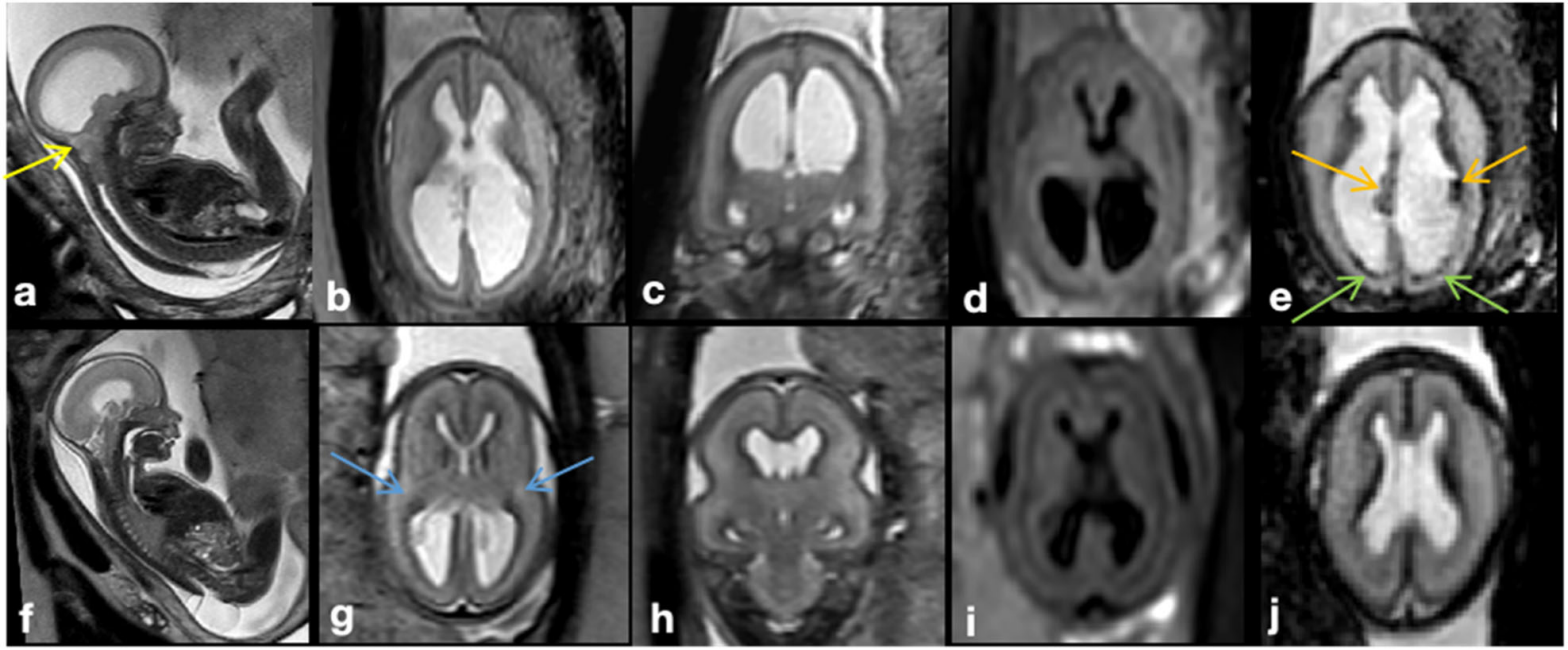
a–e MRI of a fetus with Chiari II malformation and brain edema at GW24 + 0, spinal defect formation at the lumbar level (L1/2) extending to the sacral level (S1). The posterior cranial fossa was very crowded and the vermis reached up to about C5, accompanied by protrusion of the atlantooccipital membrane (yellow arrow). b T2W-FSE images showed the compressed brain parenchyma with higher signal intensity compared to the age-matched nonedema case (without identifying the parietal crossroads) and effacement of external CSF spaces. c Blurring of lamination was shown on T2-Flair sequences. e Blood-sensitive sequences showed congested, periventricular veins (orange arrow; see Appendix Fig. 2 for postmortem MRI confirmation) and tiny hemorrhages (green arrow) along the ependyma of the posterior horn of the lateral ventricles. f–j MRI of a fetus with Chiari II malformation without brain edema at GW24 + 0. A spinal defect was found at the level of S1. f–h T2W-FSE image showed preserved external CSF spaces and distinguishable hyperintensity of the parietal crossroads in a triangle shape (blue arrows). i Normal brain lamination was shown on T2-Flair sequences. j Blood-sensitive image was normal
